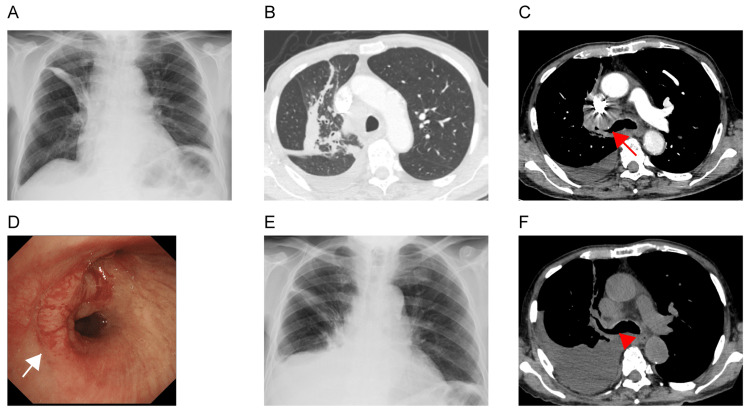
Radiological and bronchoscopic findings on the first and second admission. Chest radiograph showing band-like opacities in the right upper lung field (A). Chest computed tomography (CT) revealed a hilar tumor that had invaded and mostly obstructed the right upper lobe bronchus (arrow). Interlobar pleural effusion is present on the right side (B and C). Bronchoscopy revealed tumor invasion in the right main (arrow) and upper lobe bronchi (D). The right-sided pleural effusion increased six weeks after initiating osimertinib treatment (E). Chest CT demonstrating a decreased hilar tumor and a secured right upper lobe bronchus (arrowhead) (F).
